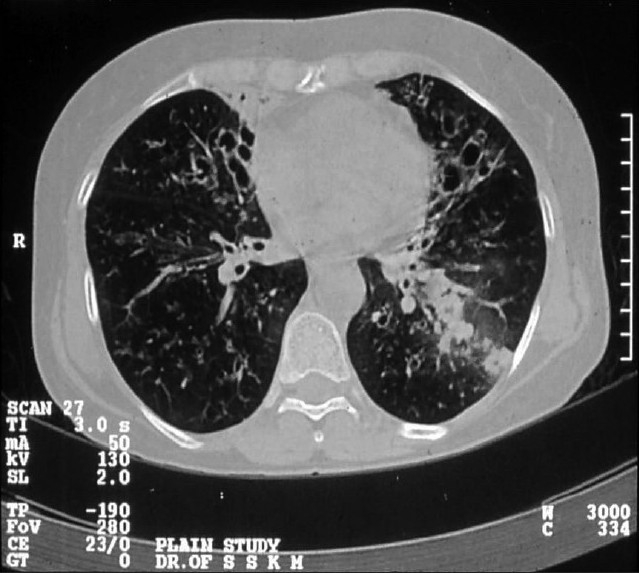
HRCT thorax showing central bronchiectasis
